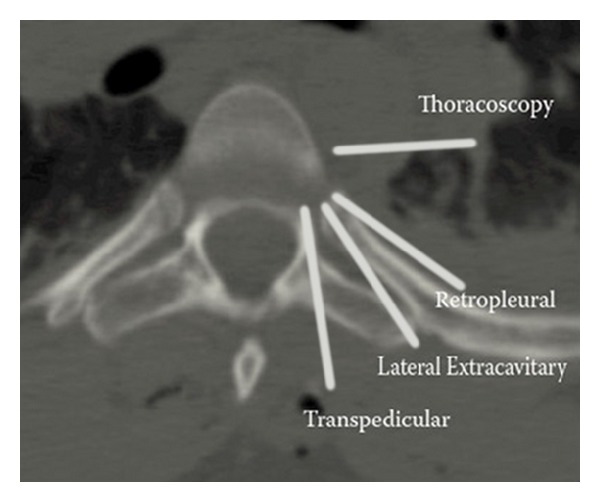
Axial CT image in midthoracic spine demonstrating the trajectory used in the various minimally invasive approaches for corpectomy.
